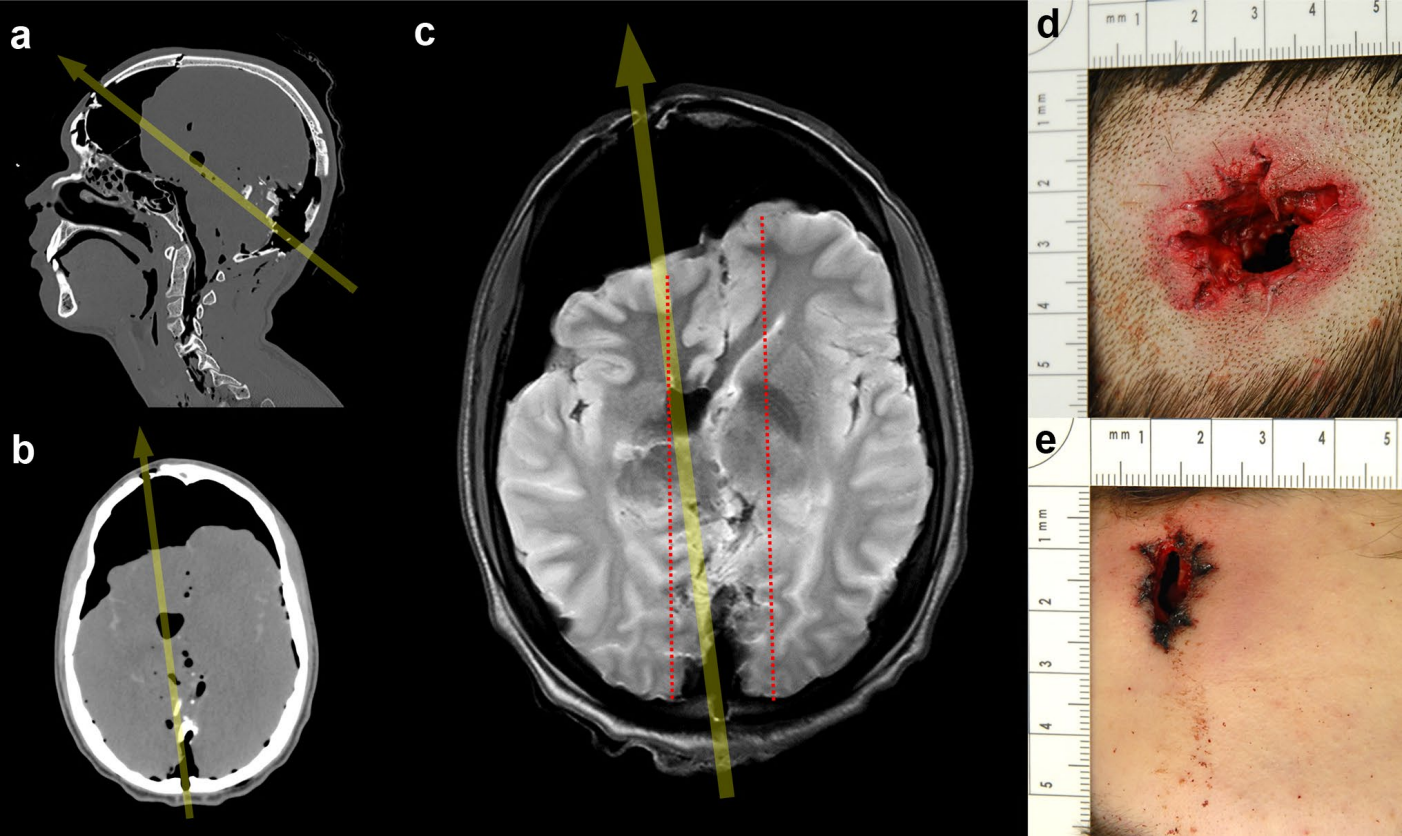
Multiplanar reconstructions of the CT data with a hard kernel (a: sagittal view), of the CT data with a soft kernel (b: transversal view), of the T2-weighted MRI data (c: transversal view), and photographs of the entrance wound, after shaving the hair, (d) and of the exit wound (e). The bullet entered the occipital bone according to the inwardly shaped bone defect and the bone fragments and exited the head at the frontal bone, resulting in an occipito-frontal trajectory (a-c: arrow). On CT images, the bullet path through the cerebral tissue was not depicted and only assumable by air bubbles (b), while MRI data clearly delineated soft tissue injuries and the wound channel (c: dotted lines)
